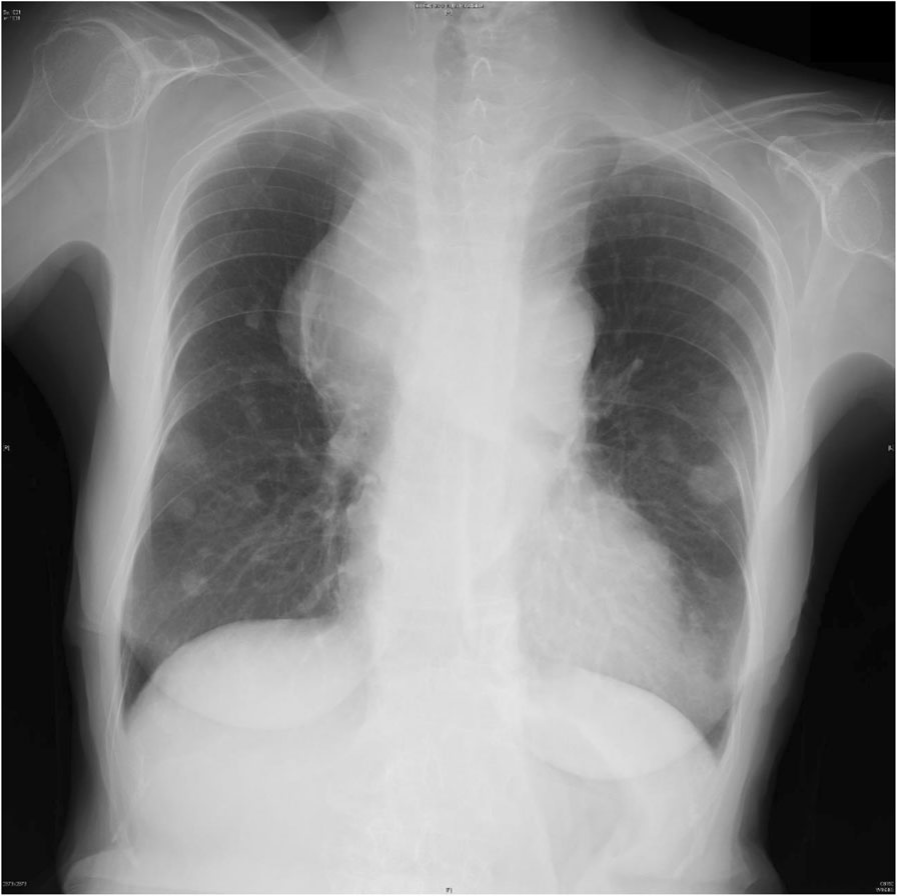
Chest X-ray before the procedure
